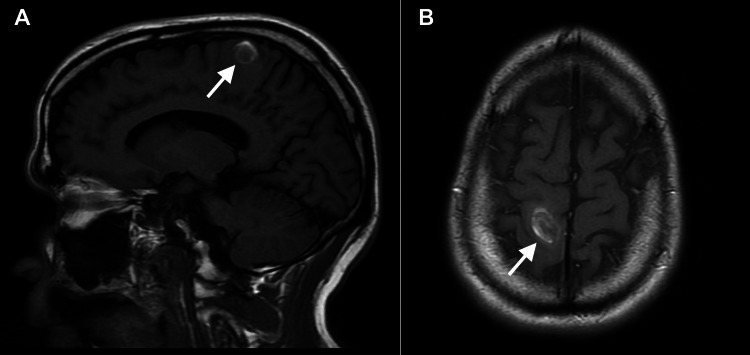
Cranial magnetic resonance imaging taken one week following presentation to the emergency department in sagittal (A) and axial (B) views
